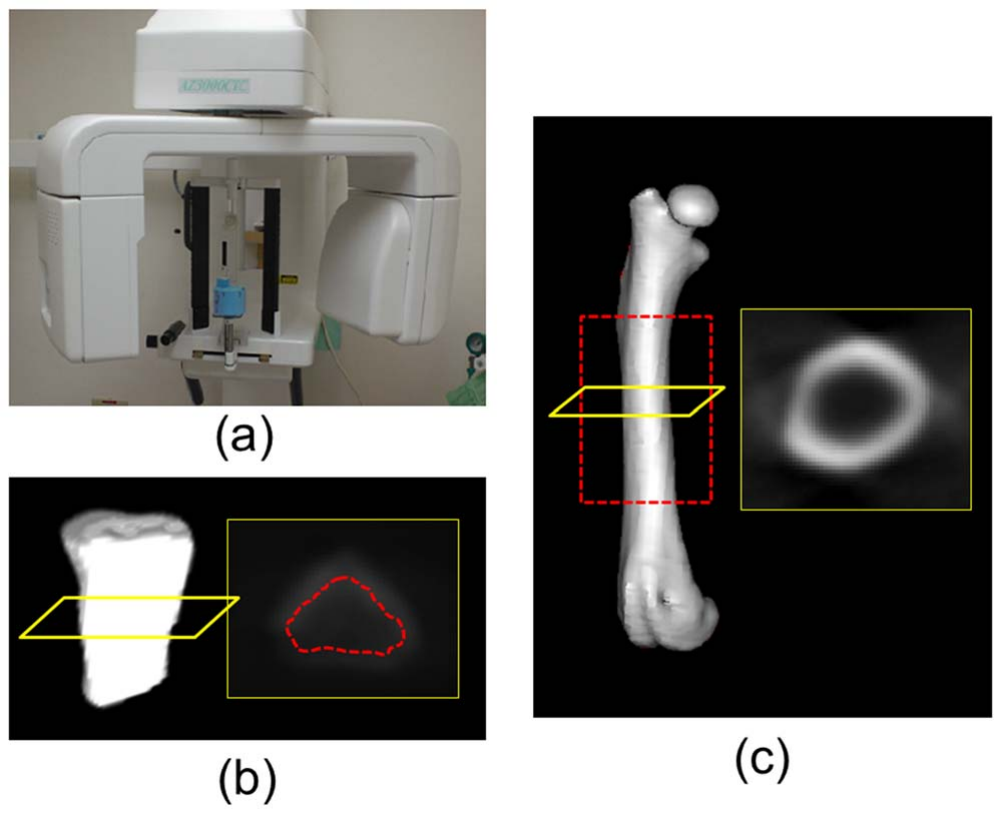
Dental CT machine, images and 3D renderings. (a) Dental CT machine (Asahi AZ 3000), (b) hemi 5th lumbar vertebral body (excluded the posterior element) in left, the section of the dental CT image in right (the region of interest for trabecular bone calculated also shown in dotted red line), and (c) intact femur (the region of interest for cortical bone calculated also shown in dotted red line) in left, the section of the dental CT image in right.
